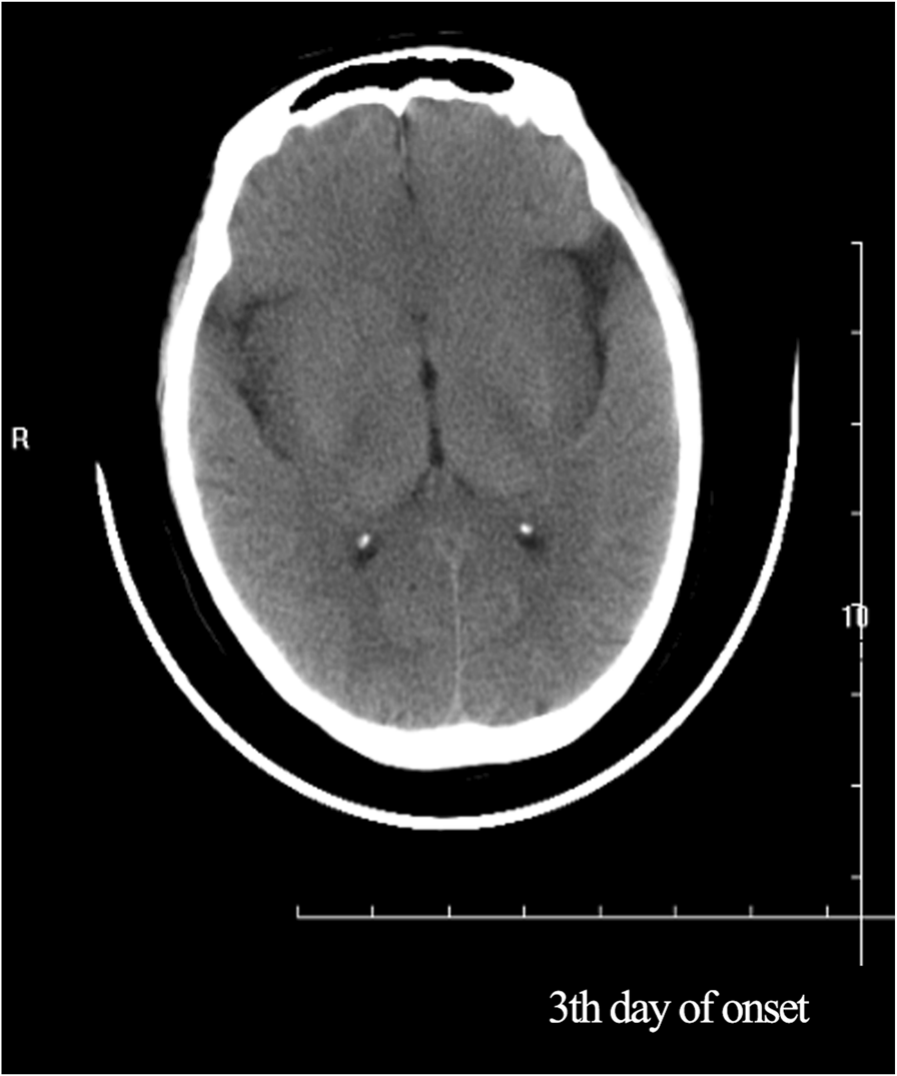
Computed tomography of the brain revealed hypodense lesions in the bilateral insula and bilateral frontal cortex, corresponding with limbic encephalitis
